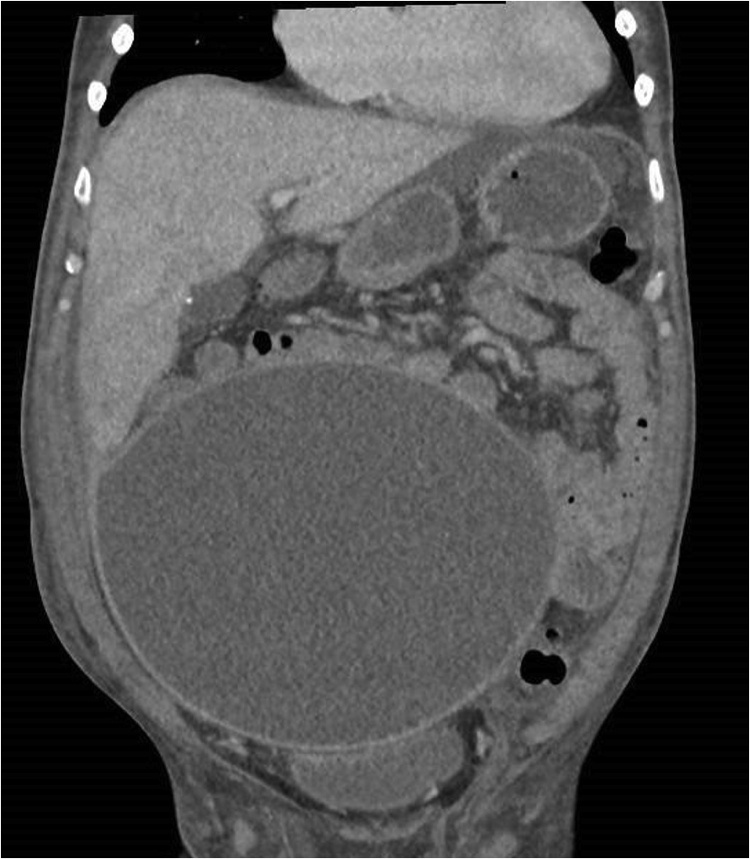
Contrast CT (coronal view) showing same lesion with a uniform wall and no abnormally thickened portion.
